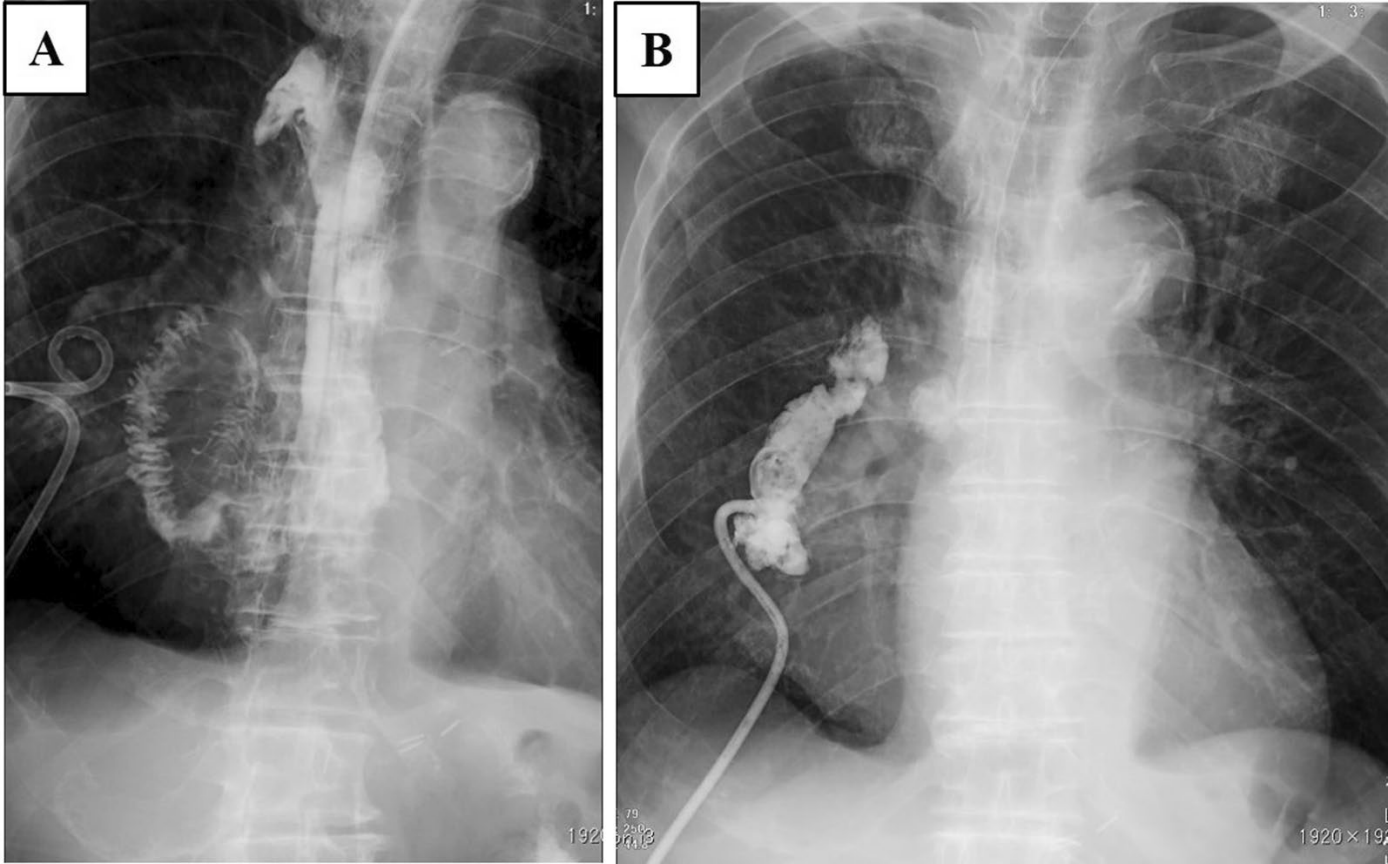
Radiographic image after the right thoracic drainage. A Esophagography revealed no evidence of perforation or interference in the passage at the reconstructed jejunum. B Fluoroscopy through the right thoracic drain showed no communication with the gastrointestinal lumen
